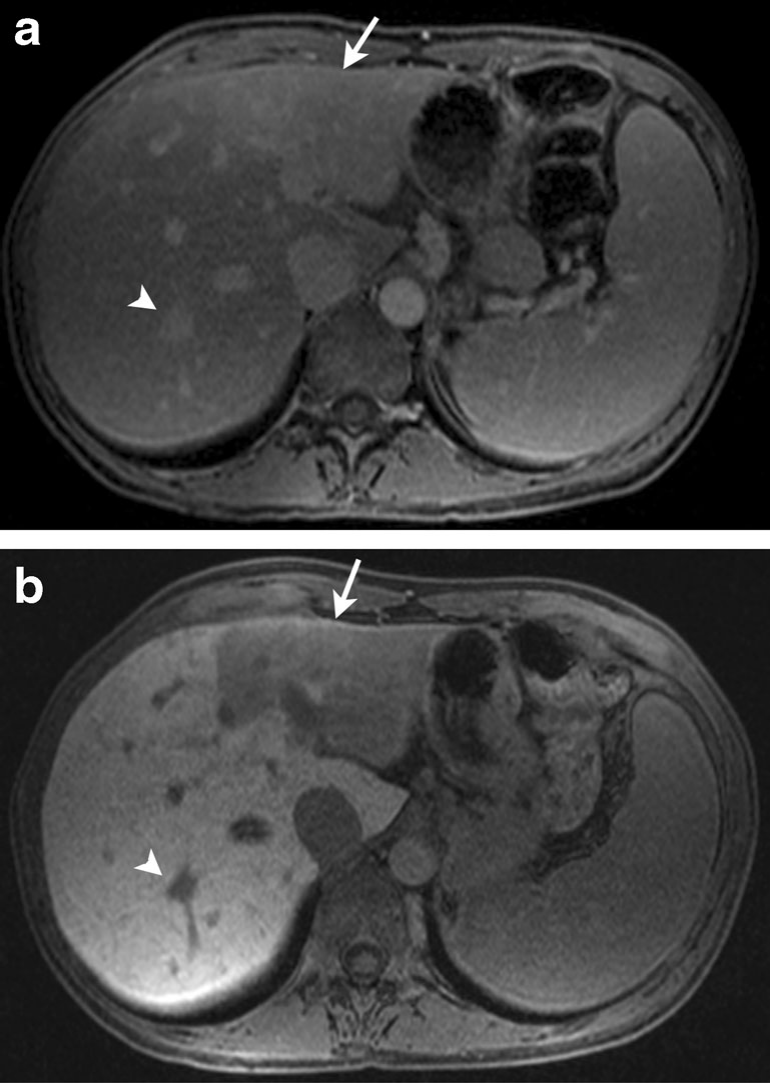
46-year-old woman with metastatic hormone receptor-positive breast cancer. a Axial fat-suppressed T1-weighted MR image in venous phase after administration of intravenous gadopentetate dimeglumine (Magnevist) shows ill-defined hyperenhancement in segment III of left lobe of liver (*arrow*) and focal hyper-enhancing lesion in segment VII of right lobe of liver. b Axial fat-suppressed T1-weighted MR image in the hepatocyte phase (20-min delay) after administration of intravenous gadoxetate disodium (Eovist) increases conspicuity of the hepatic lesions in segment III (*arrow*) and segment VII (*arrowhead*)
